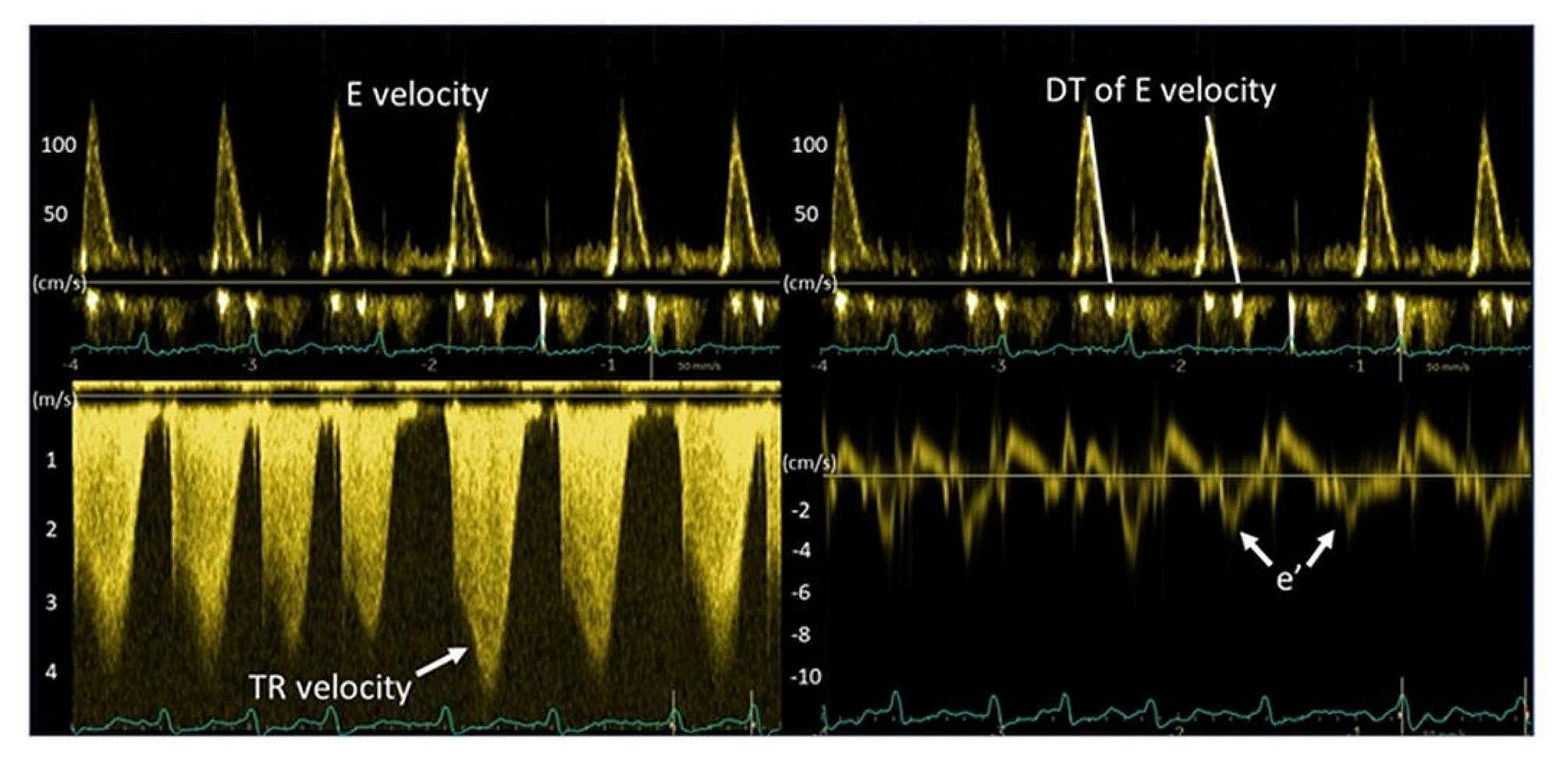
Echocardiographic recordings in a patient with elevated left ventricular filling pressure: Mitral E velocity of 120 cm/s, deceleration time of mitral E of 120 ms, peak TR velocity of 4.2 m/s, septal e’ of 3 cm/s and septal E/e’ of 40; all consistent with elevated LV filling pressure. Pulmonary capillary wedge pressure was 25 mmHg
